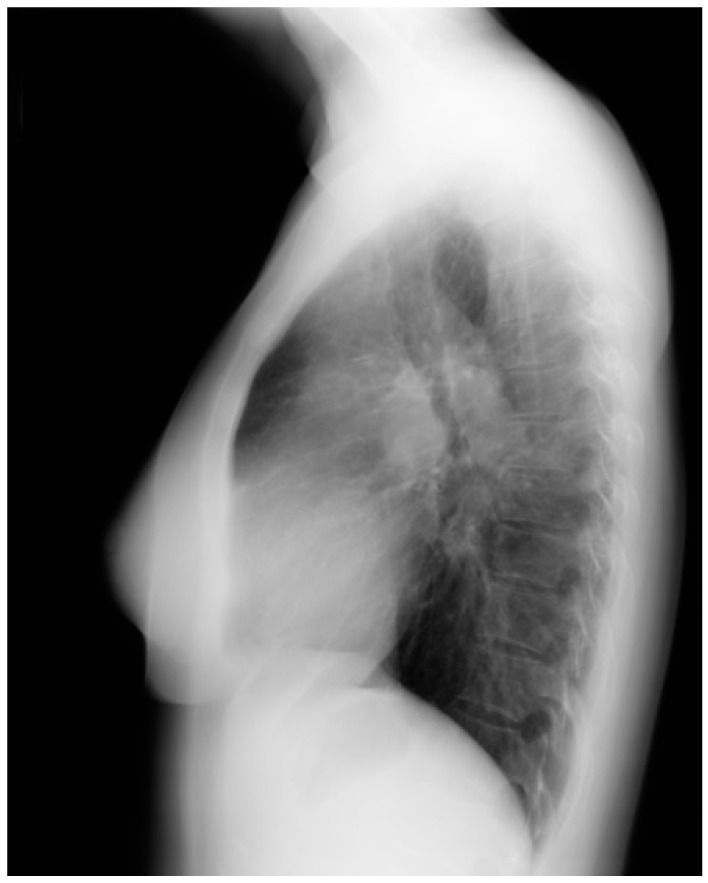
Lateral chest X-ray of patent ductus arteriosus with Eisenmenger syndrome: This is the lateral chest X-ray of the same 56-yo F with an unoperated patent ductus arteriosus (PDA) and Eisenmenger syndrome. The central pulmonary arteries are enlarged. The retrosternal airspace is encroached upon by right ventricular (RV) enlargement. Doppler echocardiography demonstrated a calcified PDA with mild right-to-left shunting, moderate RV dilatation and systolic dysfunction with severe RV hypertrophy. There was moderate pulmonary regurgitation and moderate tricuspid regurgitation. Clinically, she was NYHA Functional Class 2.
